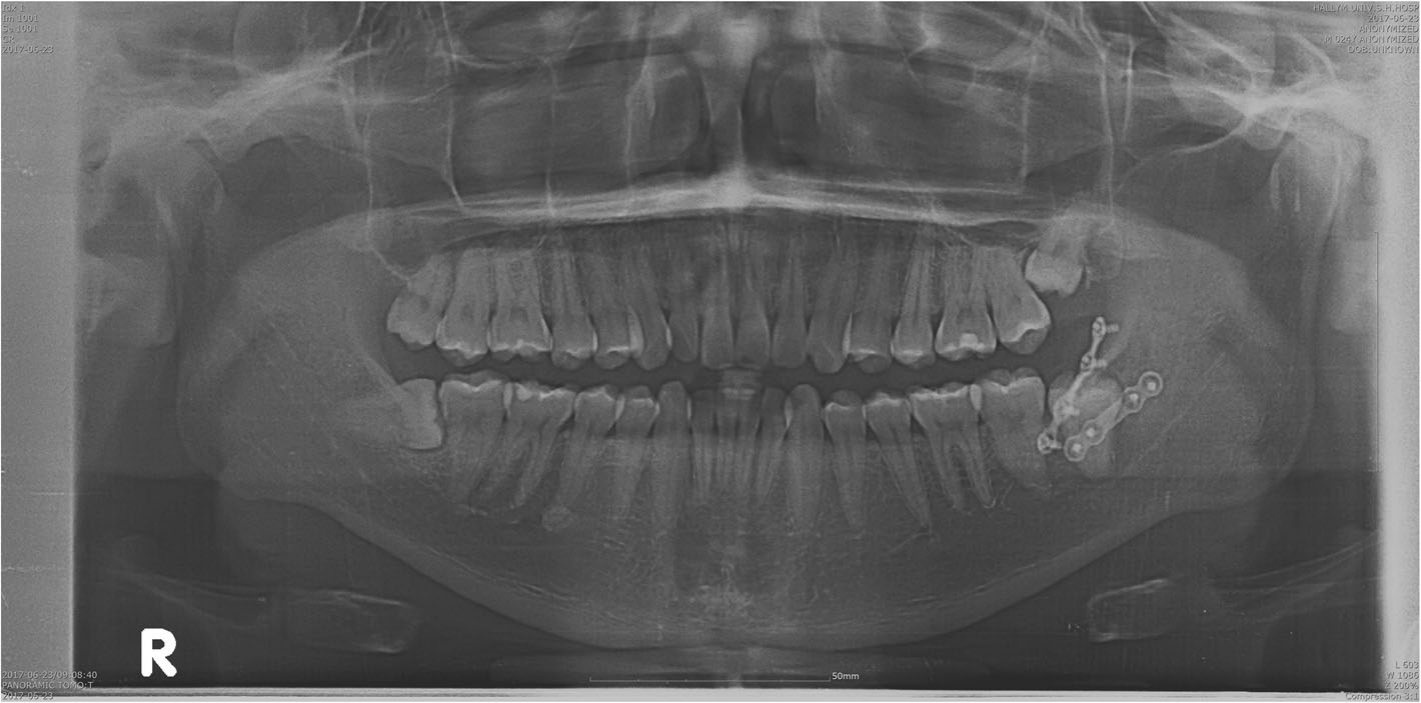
Postoperative panoramic radiographs treated with conventional plate
